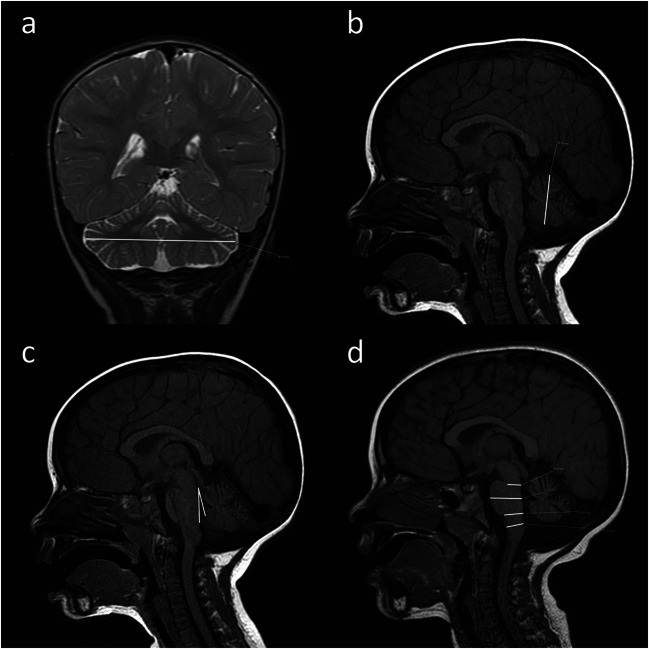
Biometry shown on brain MRI of a 7-year-old child with PMM2-CDG. a Maximum transverse cerebellar diameter (MCD). b Maximum craniocaudal height of the vermis (CCD). c Tegmento-vermian angle (TVA). d Anteroposterior measurements of the brainstem (a T2-weighted coronal image; b–d midline T1-weighted sagittal images)
